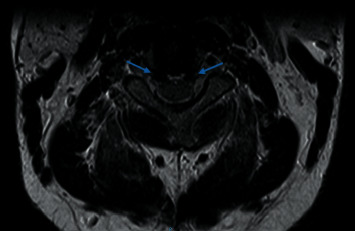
Contrast-enhanced MRI of the cervical spine, axial view. T2-weighted image: prominent epidural veins (blue arrows).
